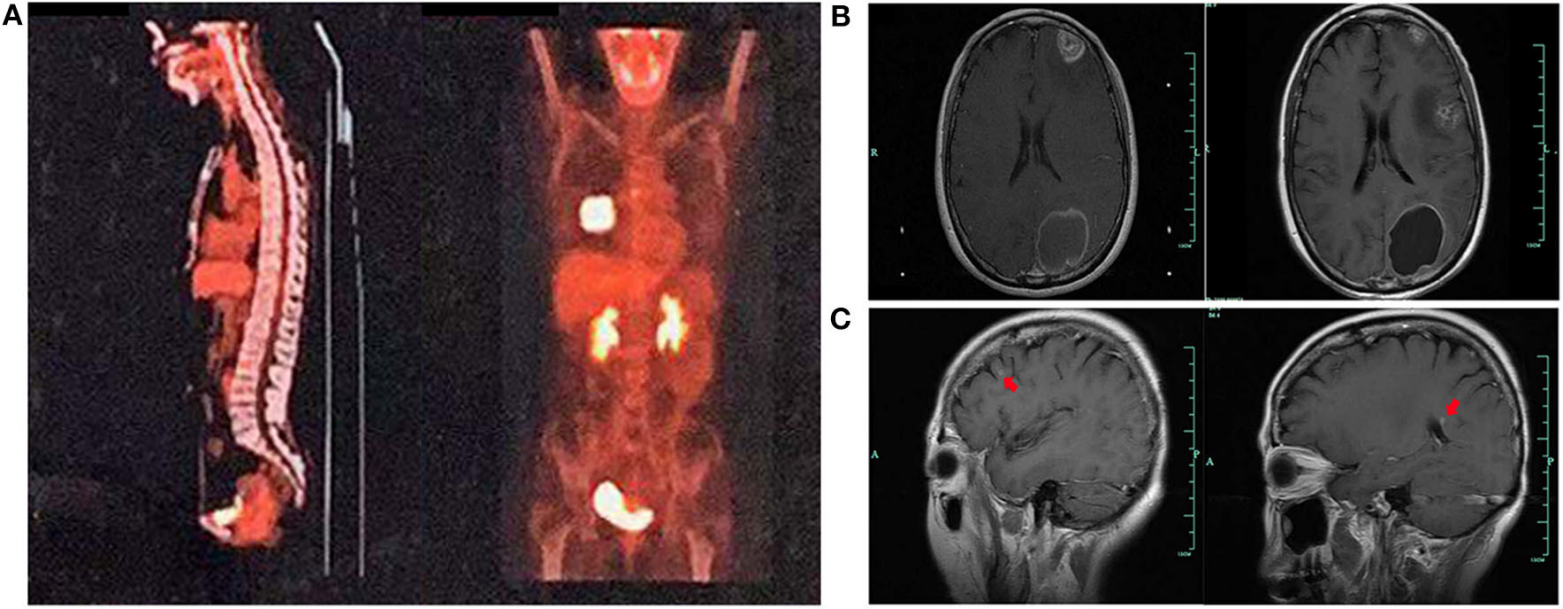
Tumor lesions detected at various times. PET-CT showing a mass in the middle lobe of the right lung (A). Tumor lesions in the brain before and after EP therapy (B). New masses in the brain after EP therapy (C).
